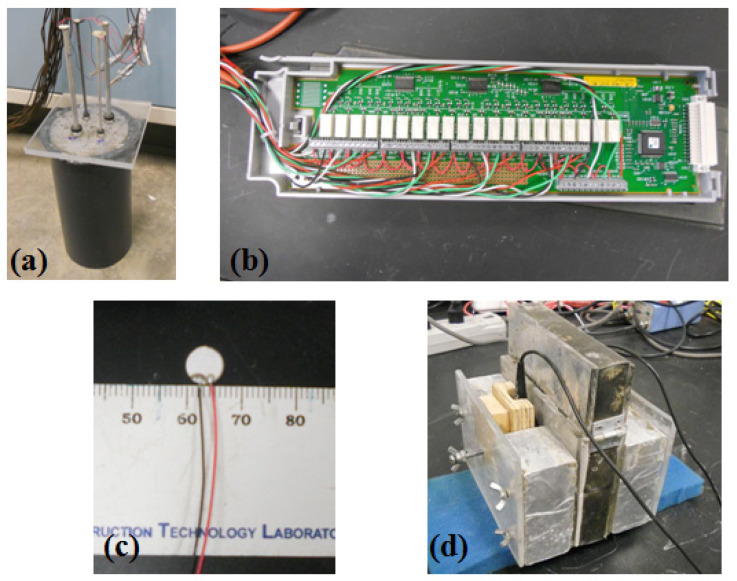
Ultrasonic Test setups: (a) guided wave testing; (b) 34903A switching card; (c) 7 mm diameter piezo disc and (d) shear wave measurement.
